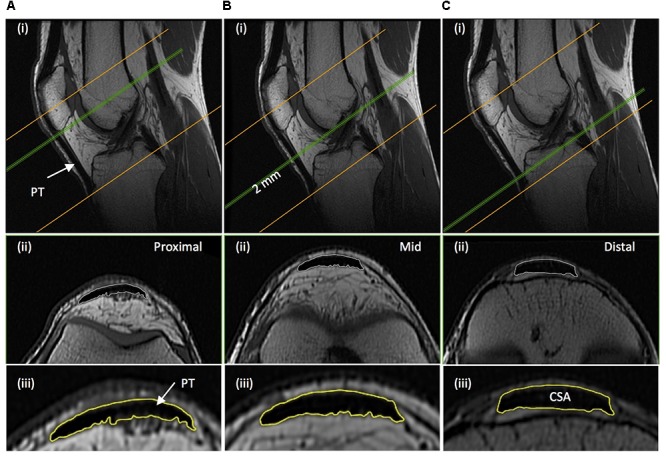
Example magnetic resonance images of the knee: (A) proximal; just distal to the apex of the patella, (B) mid-length; 50% distance between the patella-tibia attachments, and (C) distal; just proximal to the tendon tibial insertion. (i) Sagittal images show the position along the tendon length, of where the example axial images shown (ii) were acquired perpendicular to the tendon line of action. (iii) The perimeter of the patellar tendon (PT) was manually traced to determined PT cross-sectional area (CSA), with the average of the measures from each contiguous 2 mm image spanning tendon length being defined as mean patellar tendon CSA.
